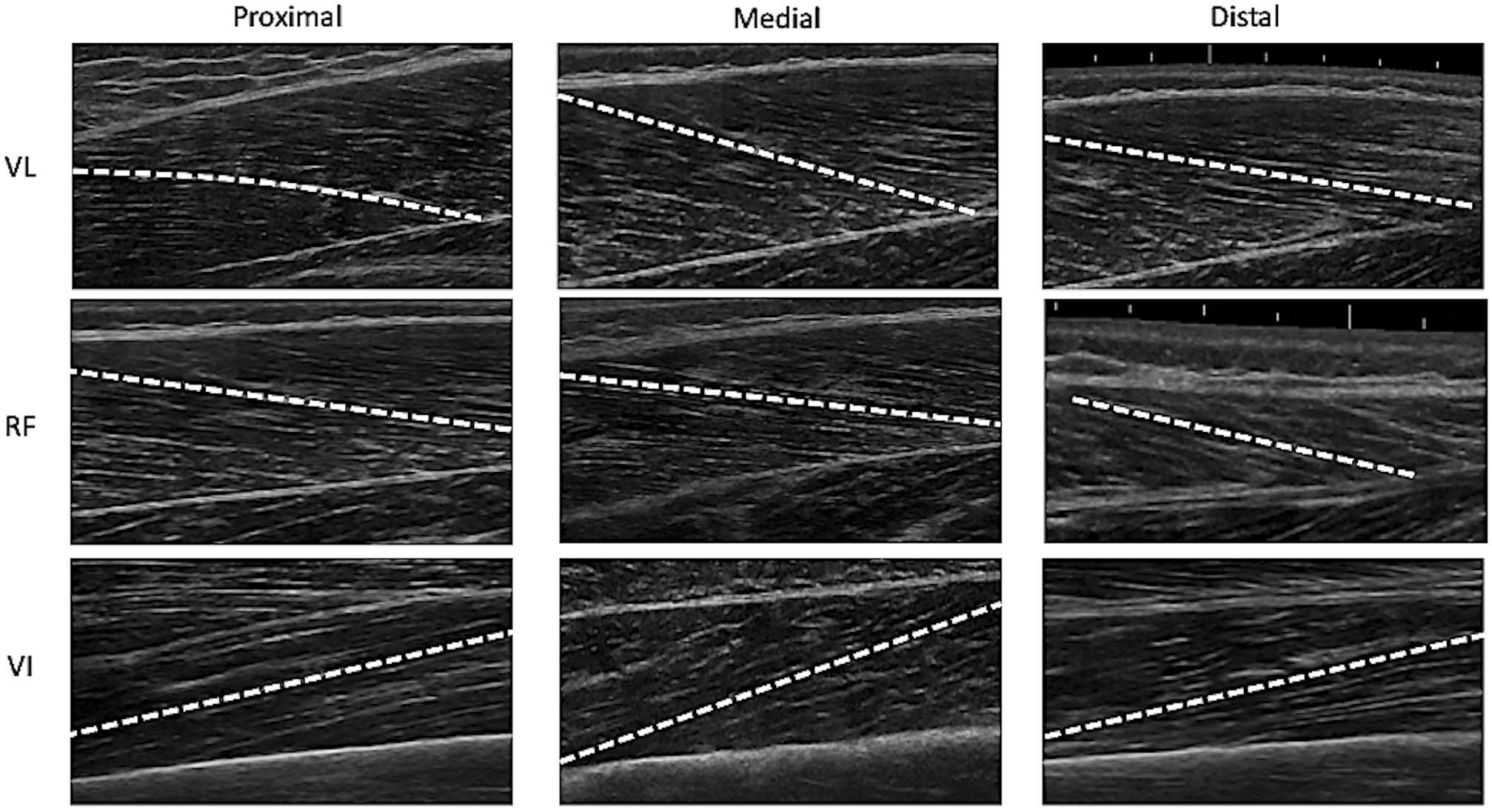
Representative ultrasound images for vastus lateralis (VL), rectus femoris (RF) and vastus intermedius (VI) in all the investigated regions
